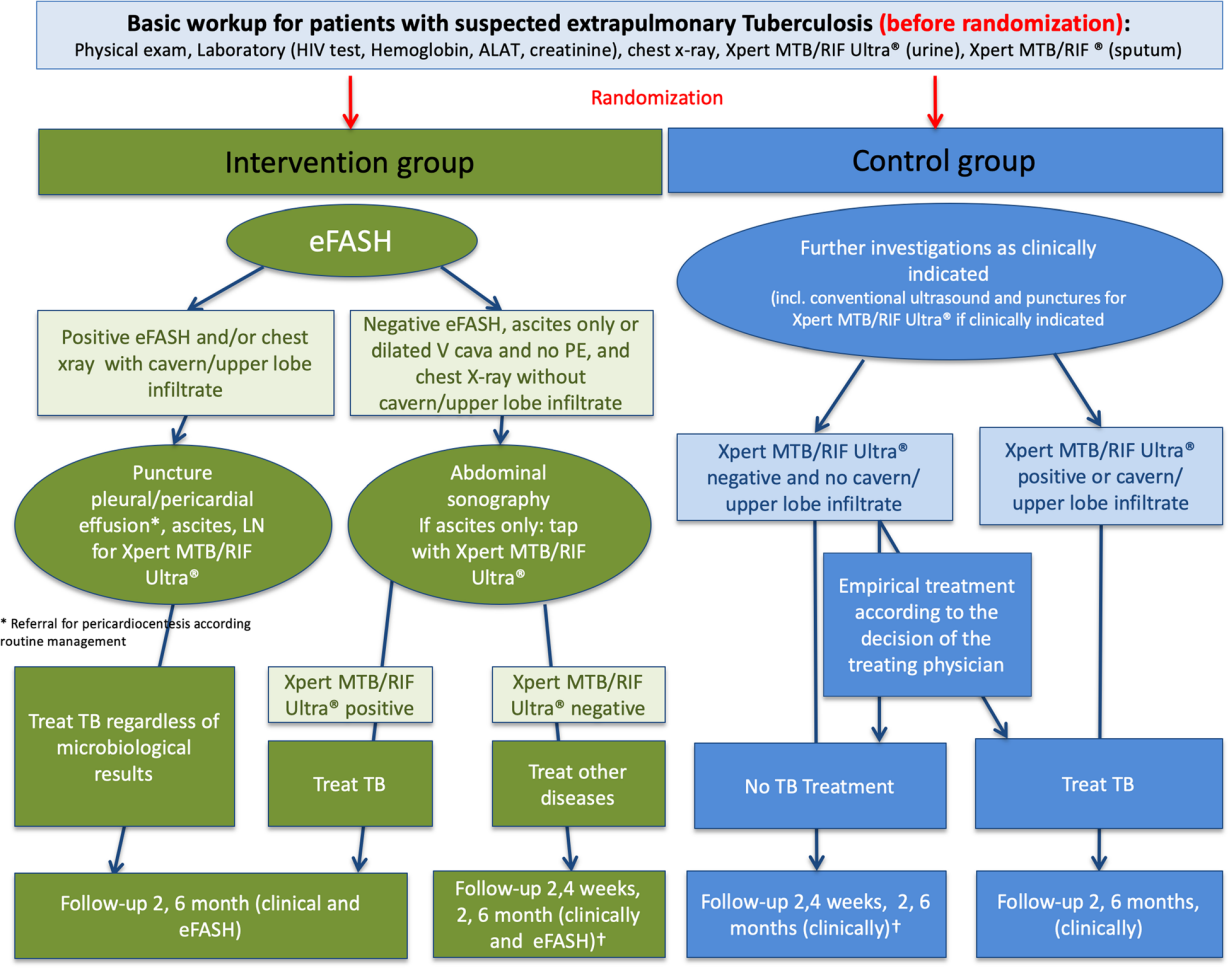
Algorithm for management of intervention group and control group. LN: lymph node; TB: Tuberculosis; eFASH: Extended Focused Assessment with Sonography for HIV and Tuberculosis; PE: pericardial effusion. † anti-tuberculosis treatment is started in case of a positive microbiological result
